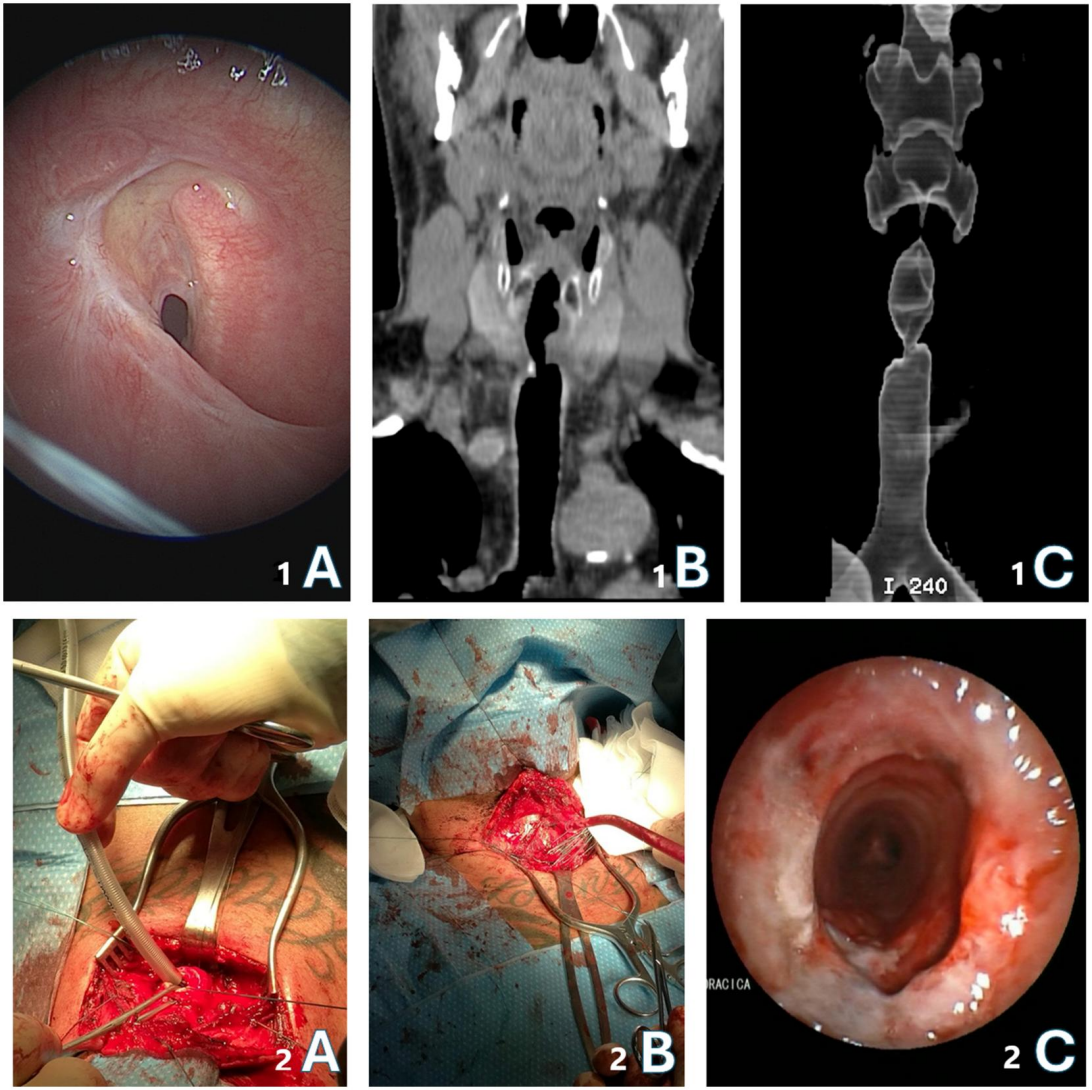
(1A) Endoscopic image of a severe subglottic tracheal stenosis; (1B) CT coronal view of the tracheal stenosis; (1C) 3D cervical reconstruction of upper airway area; (2A) intraoperative cross-field ventilation; (2B) intraoperative picture of tracheal anastomosis by single stiches; (2C) post-operative endoscopic check of trachea resection
